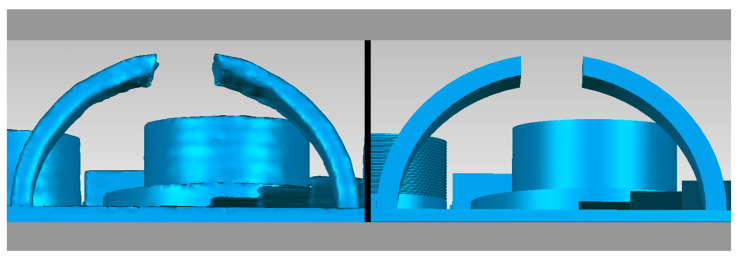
Comparison of defects in the cantilevered arc attribute ((left) CT image and (right) CAD image.
